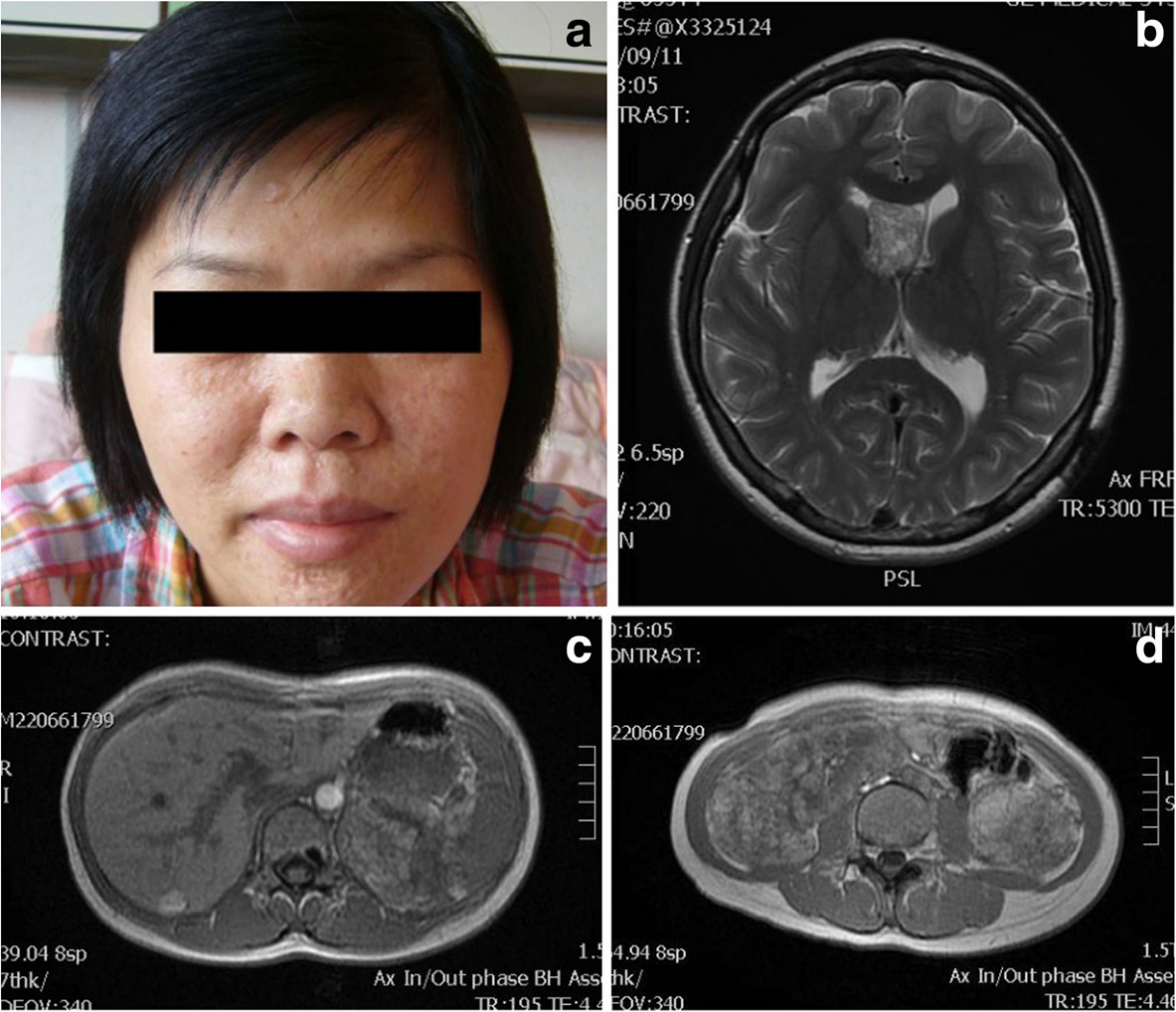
Images of the patient. a) sebaceous adenoma on the face, b) right ventricular subependymal giant cell astrocytoma, c) liver hamartoma and d) bilateral renal angiomyolipoma revealed by the magnetic resonance imaging (MRI).
